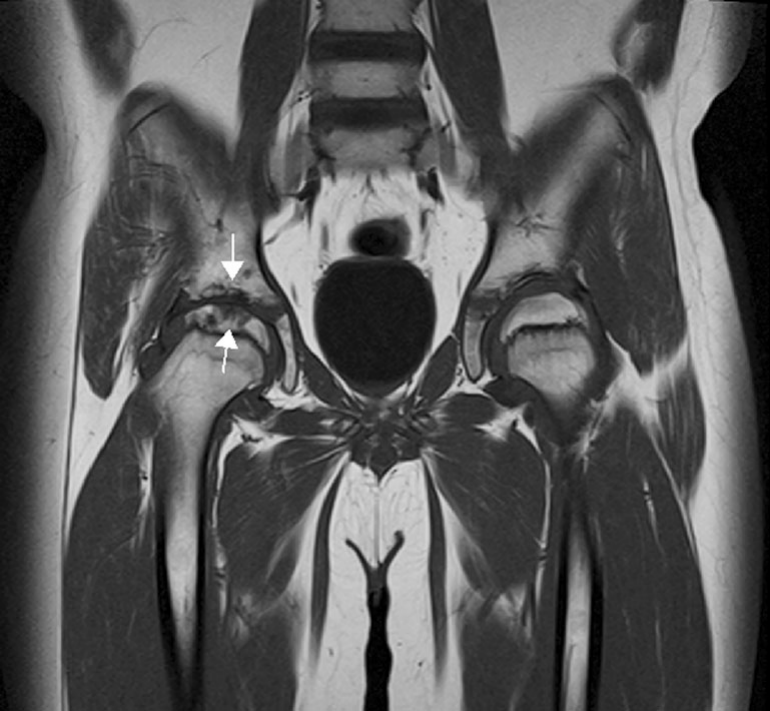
MRI can be used to depict bone destruction. Coronal T1 TSE of the pelvis shows joint space narrowing and erosions of the right hip; the same case as in Fig. 2 (arrows)
